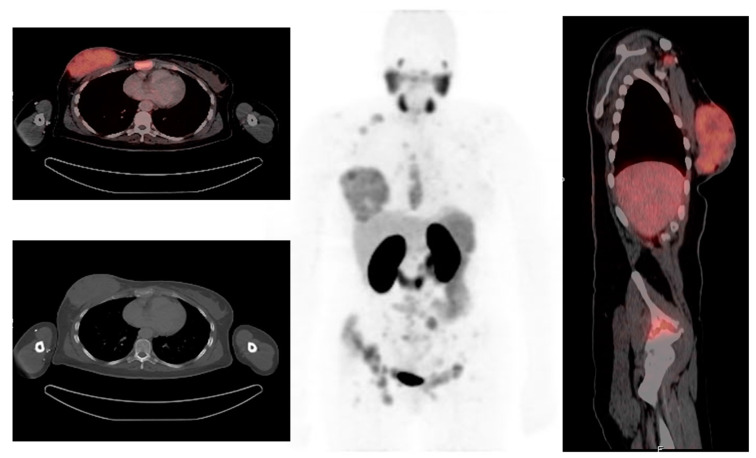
A 39-year-old woman with stage IV breast cancer underwent ^68^Ga-PSMA PET/CT. The Maximum intensity projection PET demonstrated multiple osseous metastases and primary right breast cancer. Axial and sagittal fused PET/CT confirms the ^68^Ga-PSMA avid lesions in the right breast, sternum, and right iliac bone. Images reproduced with permission from [56]; published by Eur. J. Nucl. Med. Mol. Imaging, 2017.
